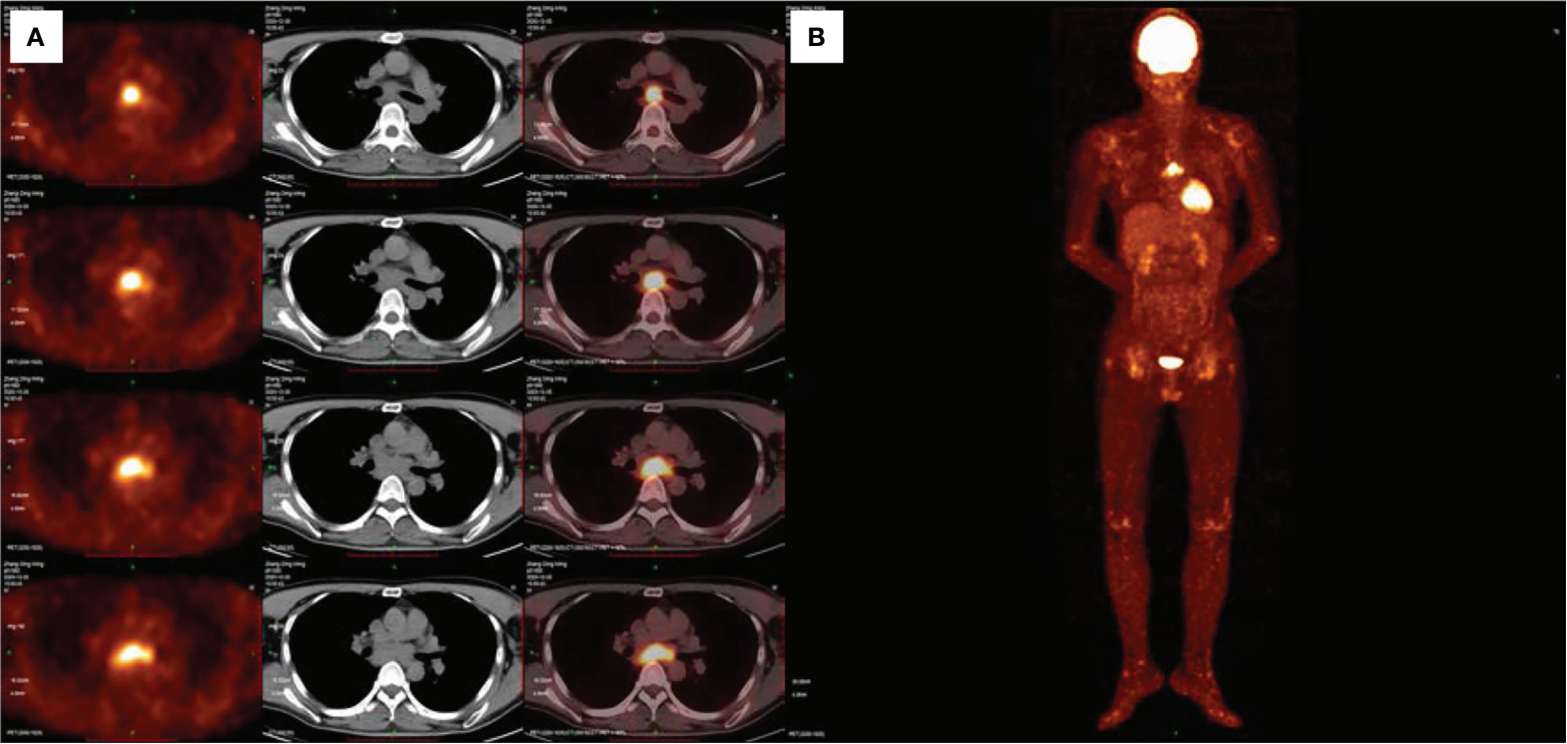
FDG-PET/CT showing increased glycometabolism in the mediastinal lymph nodes and soft tissue around the joints of the extremities (A, B).
